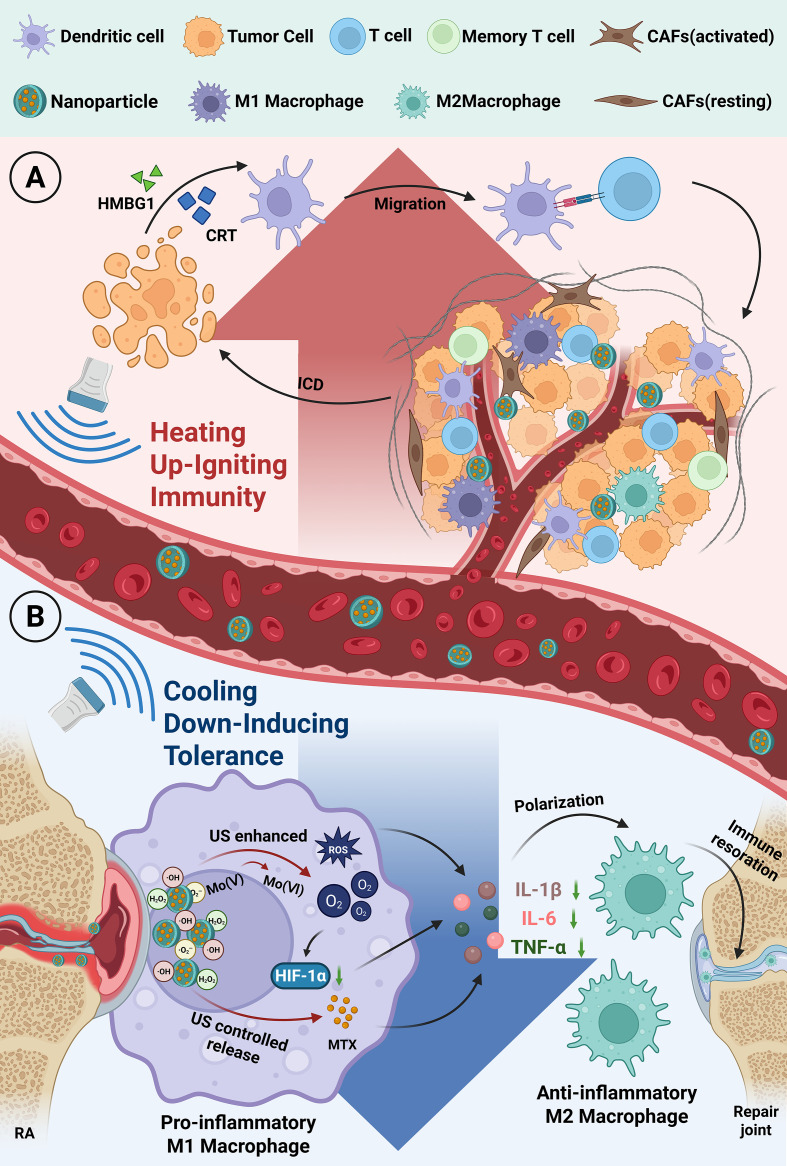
Programmability and bidirectional regulation across a spectrum of diseases (Created in https://BioRender.com): (A) Heating Up - Igniting Immunity: Ultrasound-triggered “*in situ* nanovaccines” amplify the immune activation cascade, converting “cold” tumors into “hot” immune-responsive tumors, thereby stimulating adaptive anti-tumor immunity (118). (B) Cooling Down - Inducing Tolerance: Precise delivery of nanocarriers to targeted lesions remodels the immune microenvironment, “inducing” immune tolerance and effectively driving the polarization of pro-inflammatory M1 macrophages toward the anti-inflammatory M2 phenotype, thereby alleviating the progression of autoimmune diseases (52).
